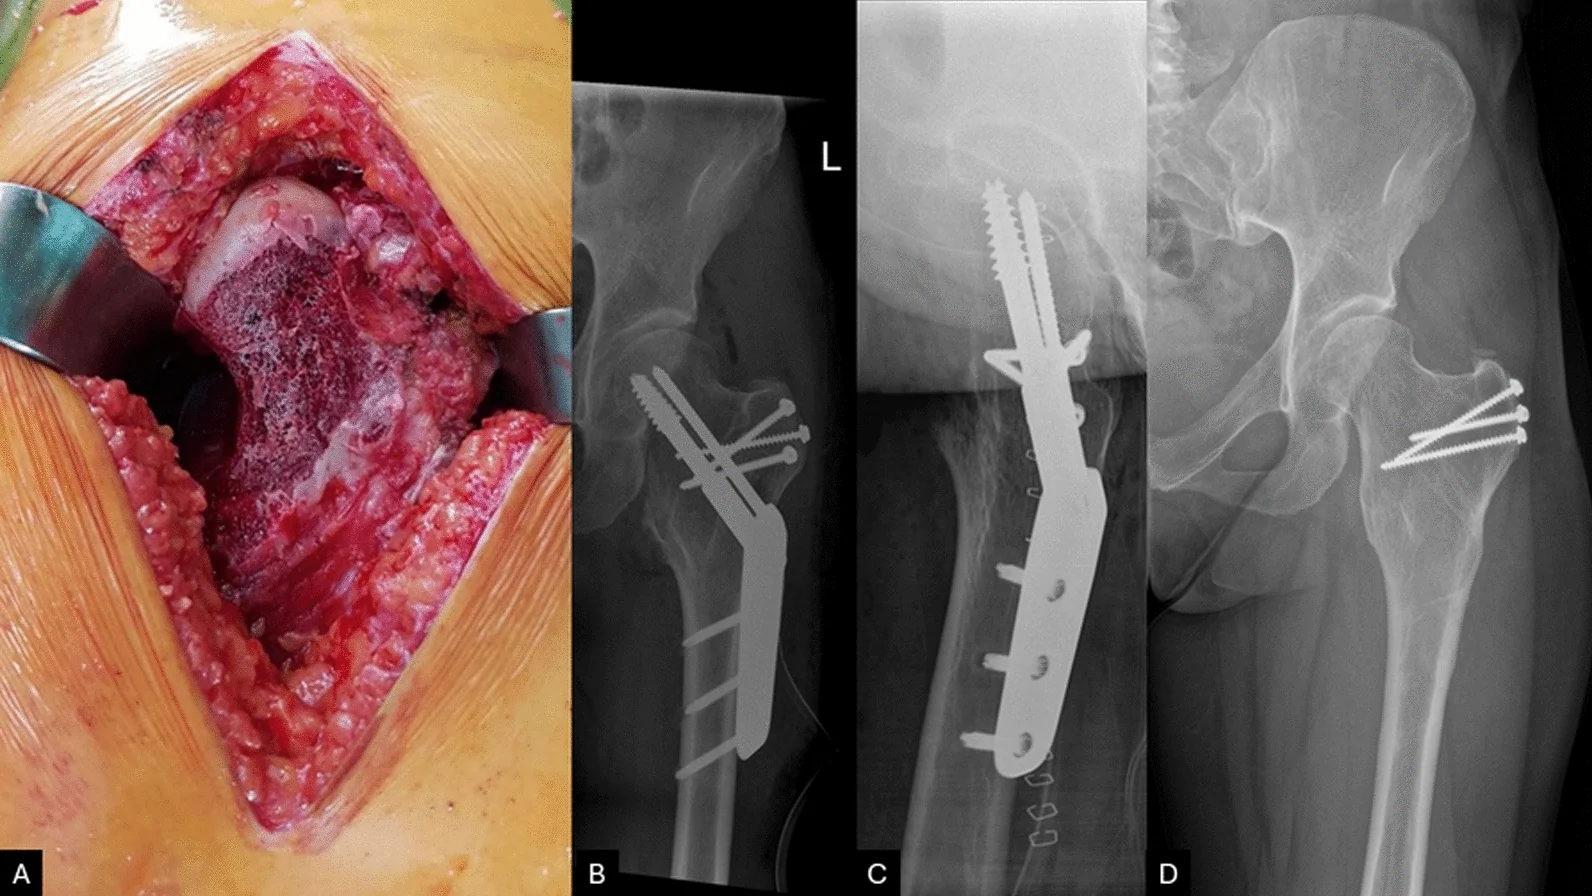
a Intra-operative image of the femoral neck following resection of the exostosis. The substance defect of the femoral neck is considerable, with almost half of the femoral neck being resected, including the cortex at the calcar region. This necessitated preventative fixation using a Dynamic Hip Screw. b Post-operative a.p. pelvis radiograph after resection of the exostosis via surgical hip dislocation and preventative fixation with an Anteversa sliding hip screw system. c Cross-table lateral post-operative radiograph highlighting the extent of the resection undertaken. d An anteroposterior radiograph of the pelvis, taken seven years after the initial surgery, reveals healing and remodelling of the femoral neck and trochanteric region; implant removal was carried out approximately 18 months after the initial procedure
